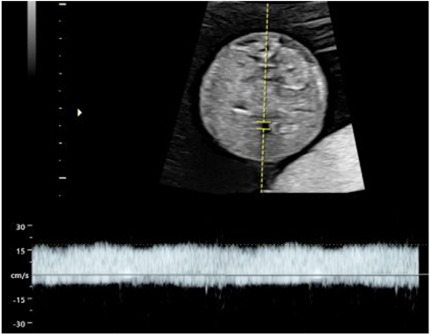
Flow: spine up/down; pulsed-wave Doppler in mid-abdominal portion; insonation angle ≤30° (ideally 0°).
